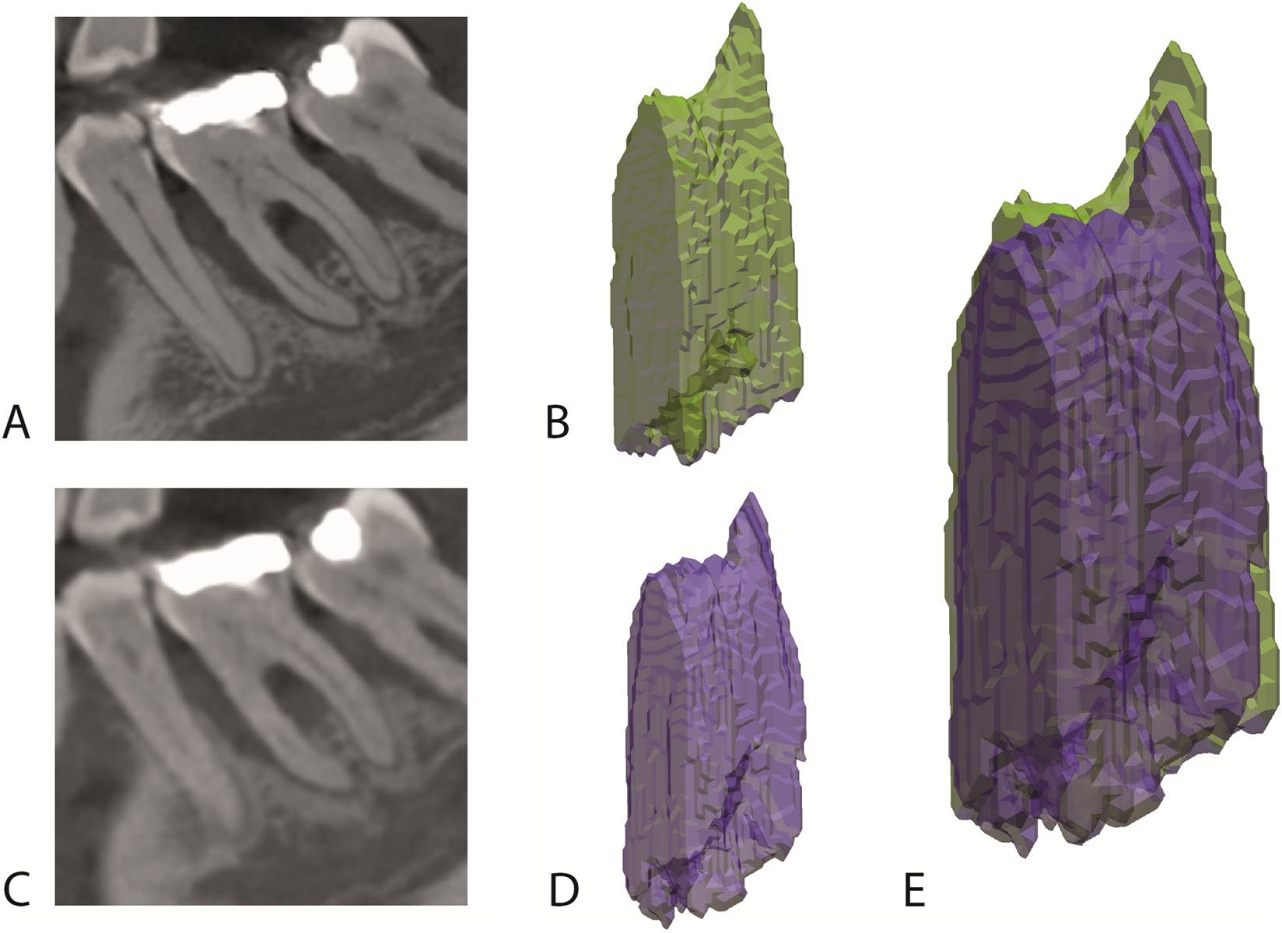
Volume segmentation of the furcation at tooth 36. (A) HD-CBCT of tooth 36 in the sagittal plane. (B) Corresponding volume model after segmentation and export as STL data into Geomagic Design X. (C) LD-CBCT of tooth 36 in the sagittal plane. (D) Corresponding volume model after segmentation and export as STL data into Geomagic Design X. (E) Overlay of the two STL data sets showing the high volume agreement between HD-CBCT and LD-CBCT.
